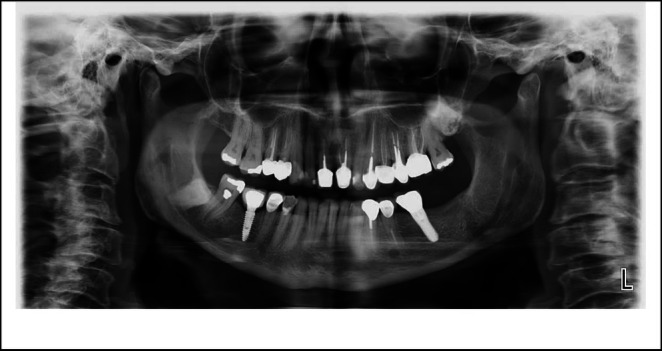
One‐year postoperative panoramic radiograph. The patient was asymptomatic.
